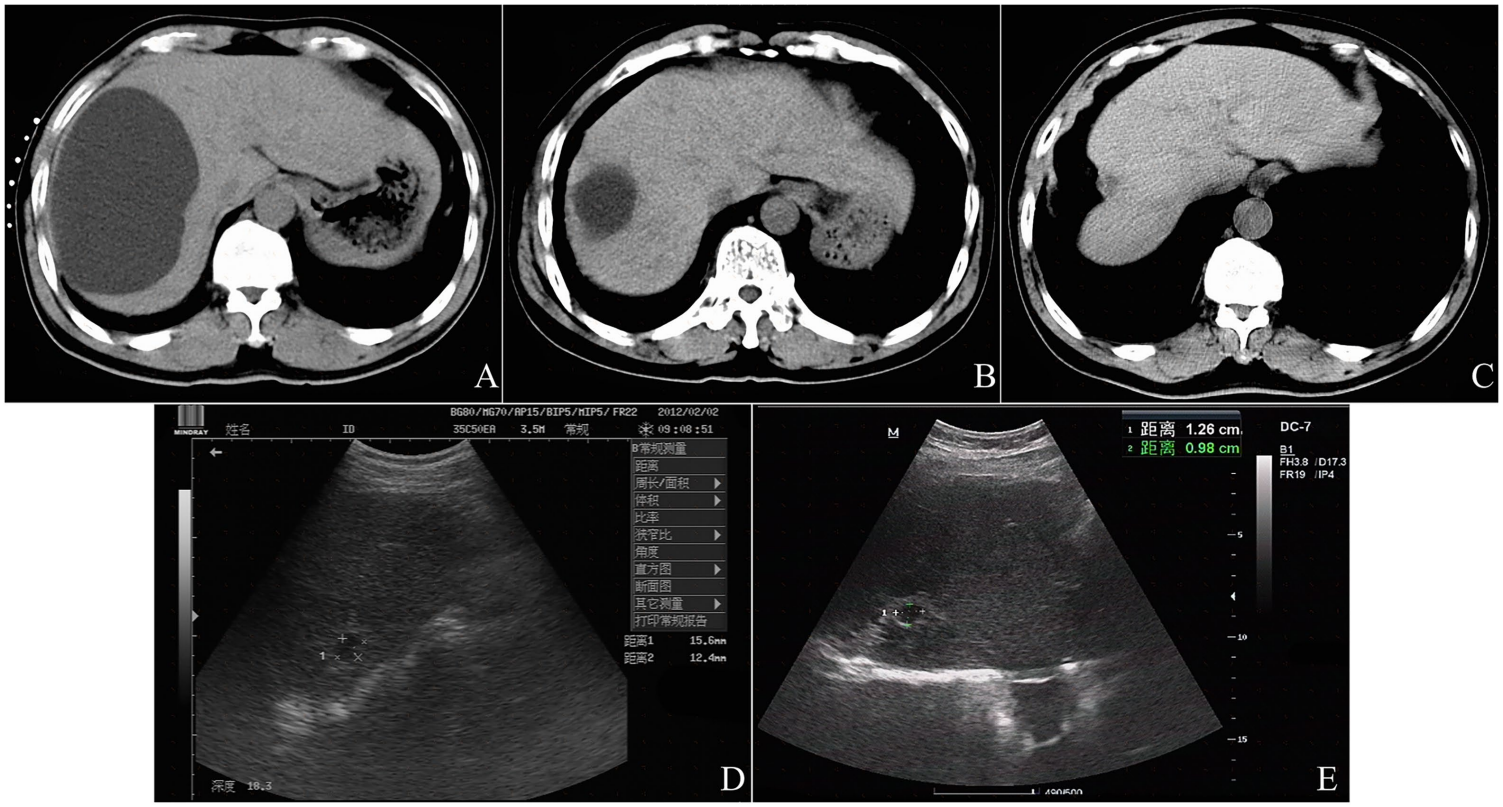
A typical example of the imaging-based treatment response after CT-guided percutaneous aspiration and bleomycin sclerotherapy in a 69-year-old man with a simple left renal cyst. Preprocedural CT scan shows an initial cyst volume of 554.4 mL (12.6 cm × 8.8 cm × 10.0 cm in size) (A). At 6-month follow-up, CT shows a cyst volume of 34.3 mL (4.9 cm × 3.5 cm × 4.0 cm in size) and the volume reduction rate (VRR) of 93.8% (B). At 12-month follow-up, CT shows a cyst volume of 1.7 mL (20.0 mm × 13.3 mm × 13.0 mm in size) and the volume reduction rate (VRR) of 99.7% (C). At 24-month follow-up, US shows a cyst volume of 1.2 mL (15.6 mm × 12.4 mm × 13.0 mm in size) and the volume reduction rate (VRR) of 99.8% (D). At 36-month follow-up, US shows a cyst volume of 0.7 mL (12.6 mm × 9.8 mm × 12.0 mm in size) and the volume reduction rate (VRR) of 99.9% (E).
